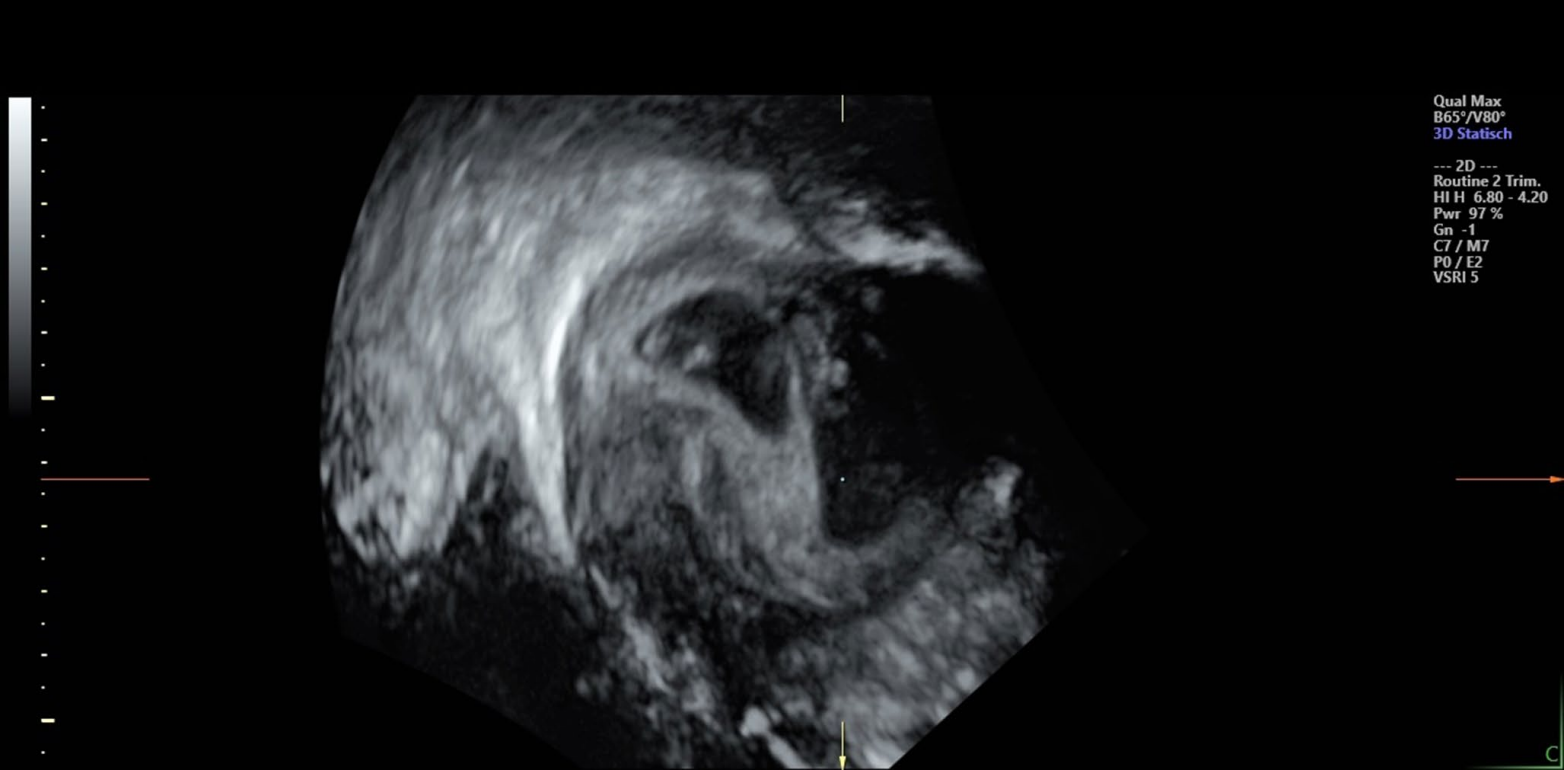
Ultrasound images from the 10th week of a dichorionic diamniotic twin pregnancy, where the twins are separated by a thick layer of fused chorionic membranes. Patient permission has been granted
